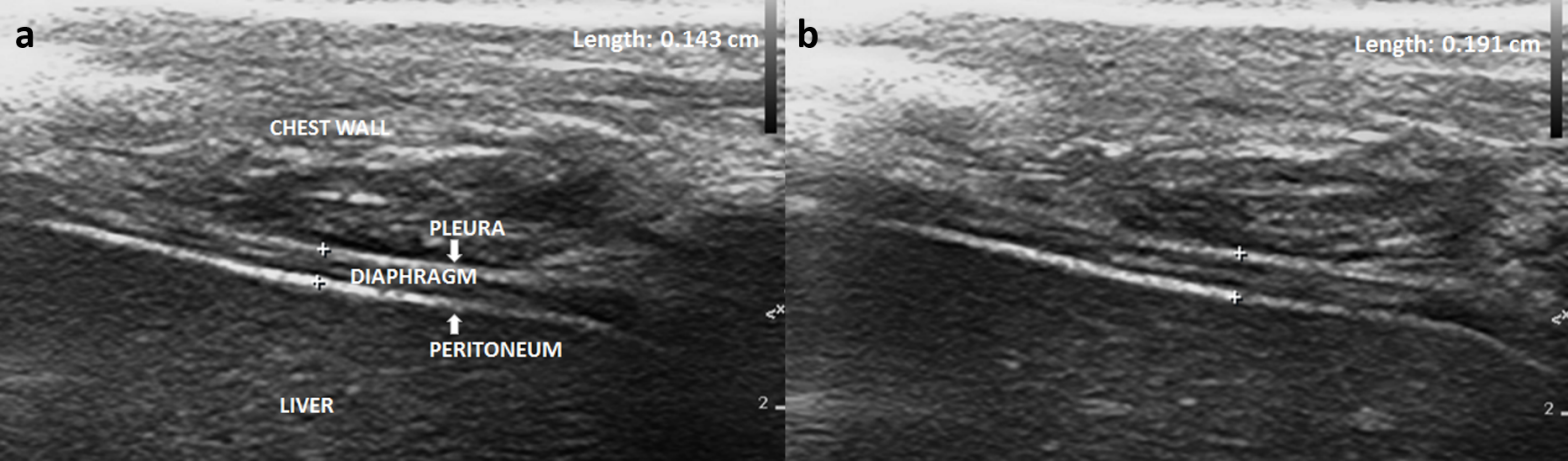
Diaphragm ultrasound at the zone of apposition. (a) Thickness measurement at end-expiration, (b) Thickness measurement at end-inspiration.
